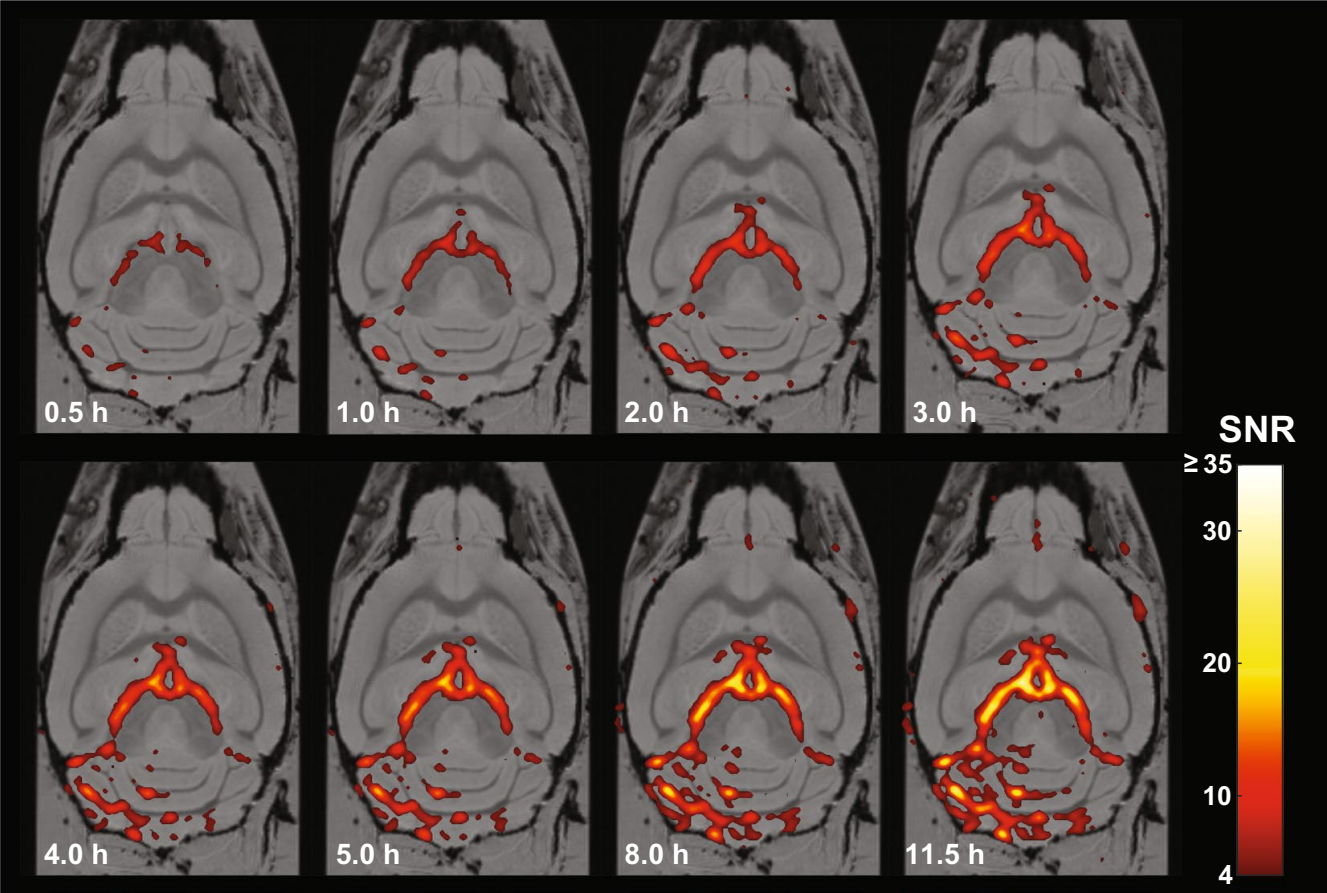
High spatial resolution ^19^F MRI using acquisition times feasible for *in vivo* imaging. ^19^F MR images were acquired with the ^*19*^
